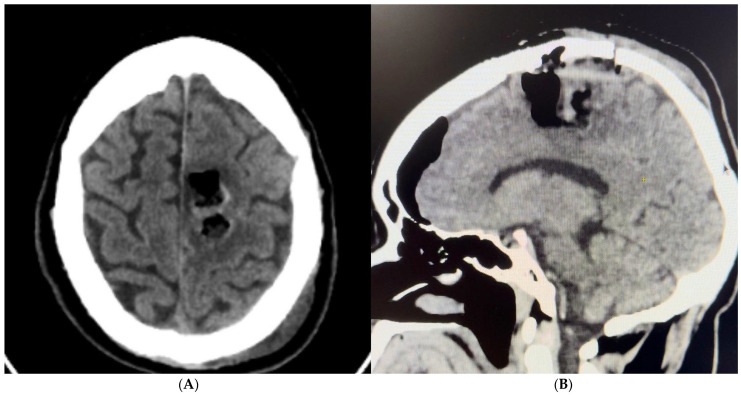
(A,B) Postoperative CT scan confirmed surgical changes and complete resection of the lesion.
